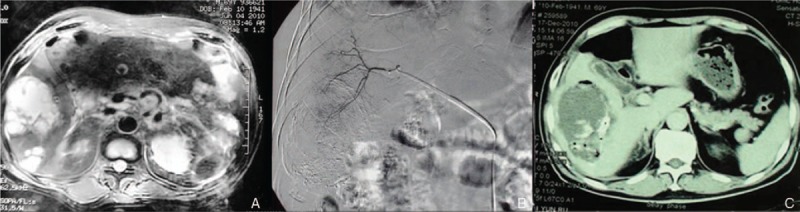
Liver abscess after the treatment of TAE. (A) MRI scan before TAE; (B) image during the treatment of TAE; (C) CT scan after TAE, which showed the occurrence of liver abscess. CT = computed tomography, MRI = magnetic resonance imaging, TAE = transcatheter arterial embolization.
